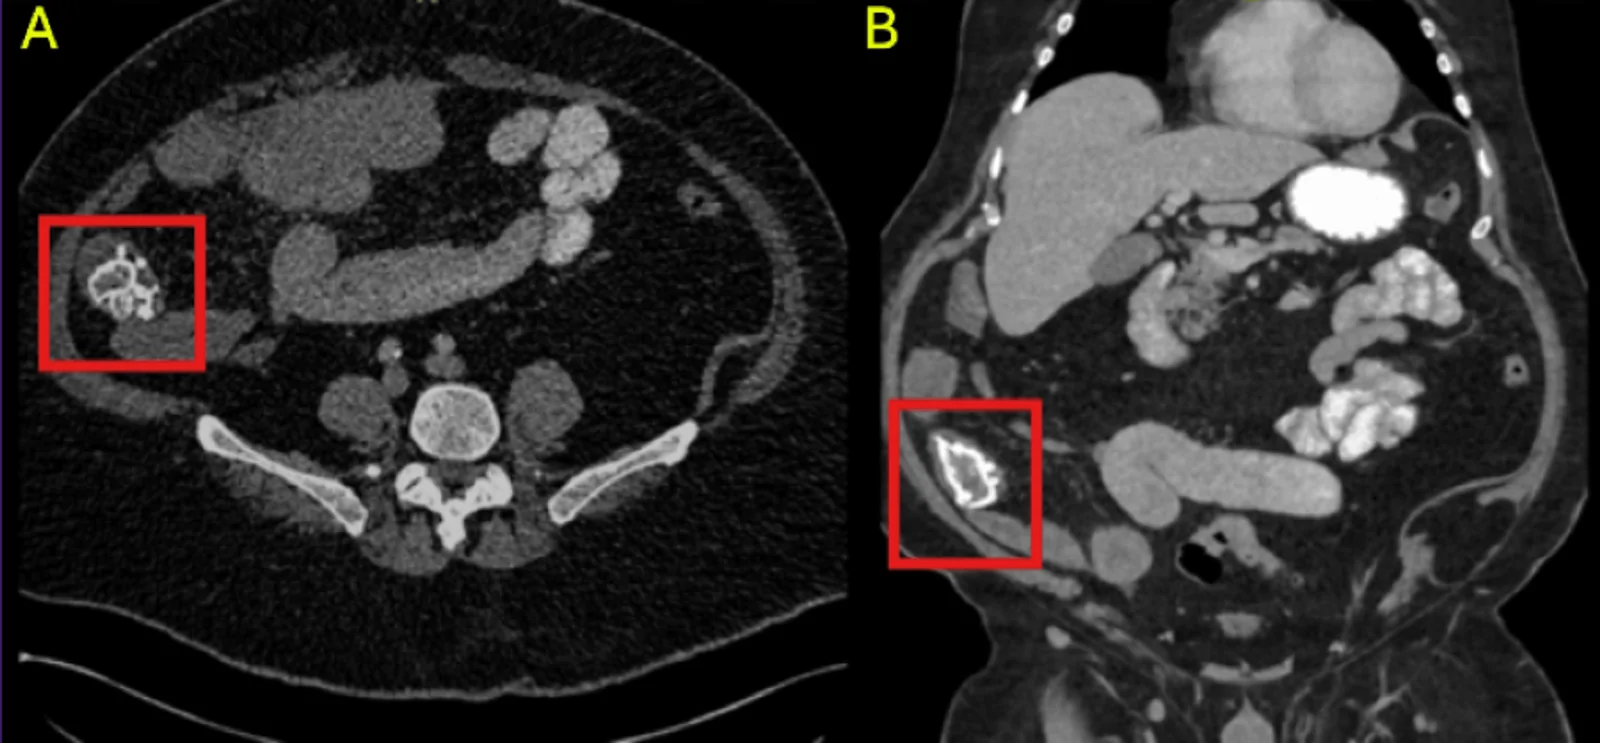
CT of the abdomen and pelvis demonstrated a small bowel obstruction with an intraluminal foreign body in the distal ileum A: Axial view, B: Coronal view
